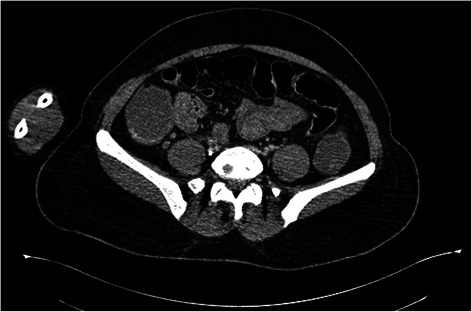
Abdominal computed tomography of the abdomen showing an irregular wall thickening
